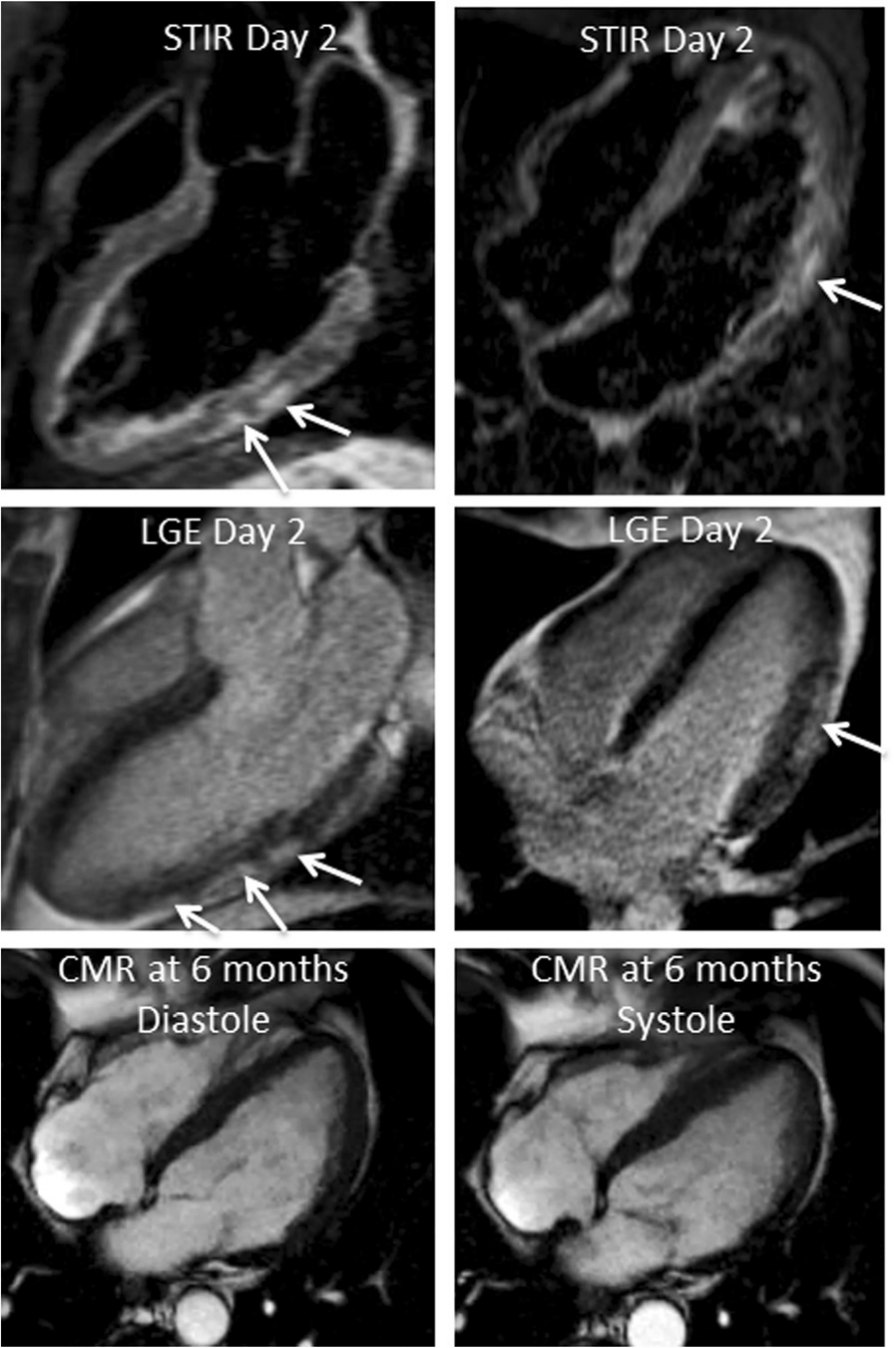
CMR data findings of a 56-year-old male performed at day 2 after the onset of an acute coronary-like syndrome (chest pain, ST segment alterations, mild troponin elevation). Black blood T2-weighted STIR CMR indicates the presence of limited subepicardial hypersignal in the mid portion of the posterolateral and lateral LV walls, indicative of small foci of myocardial edema (top panel, arrows, 4 % LV mass). Late gadolinium-enhanced CMR shows limited subepicardial nodular lesions in the mid portion of the posterolateral and lateral LV walls (mid panel, arrows, 6 % LV mass), indicative of acute myocarditis. Cine-MR at day 2 and 6 months (bottom panel) showed altered systolic global LV function (LV end-diastolic volume index 83 ml/m^2^, LV ejection fraction 45 %; 101 ml/m^2^ and 36 %, respectively). Despite optimal medical therapy, the patient suffered NYHA Class III heart failure during follow-up with diffuse LV hypokinesia predominant in the lateral wall
